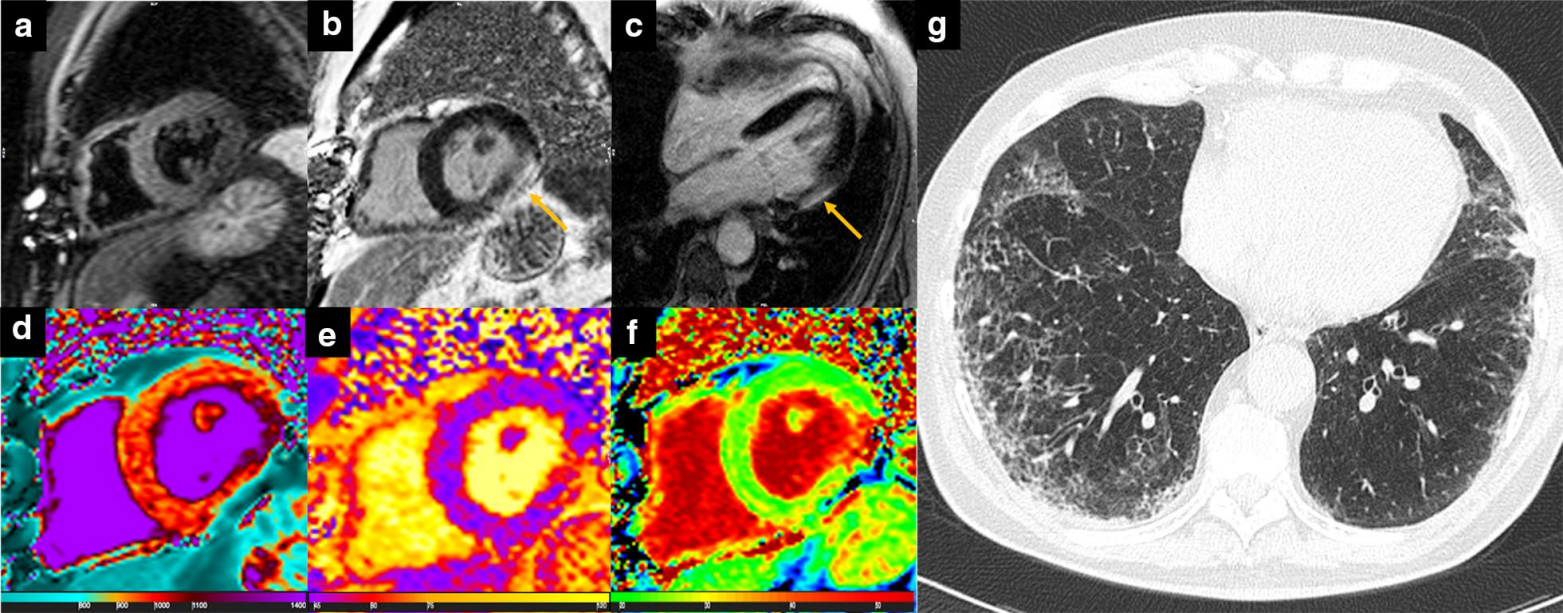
Myocarditis. 51-year-old man hospitalized for COVID‐19 pneumonia and sudden onset of tachyarrhythmias. STIR images revealed no edema (a), although LGE was evident on infero-lateral segments of basal-mid planes with a subepicardial pattern of distribution (b, c orange arrows) and native T1 was increased on LGE + segments (d). T2 mapping sequences revealed the presence of edema on infero-lateral segments of mid-ventricular planes (e). ECV confirmed those findings with implemented values on infero-lateral wall (f). Chest CT showed GGO predominantly distributed on inferior lobes with a peripheral distribution (g). *STIR* short tau inversion recovery, *LGE* late gadolinium enhancement, *ECV* extracellular volume, *CT* computed tomography, *GGO* ground glass opacity
